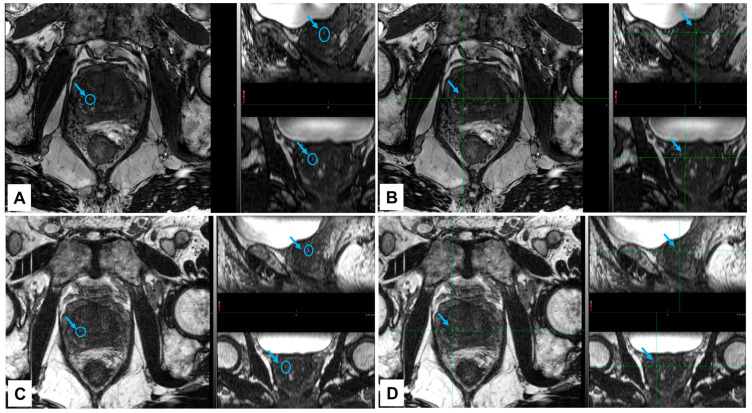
Visibility of NOVA fiducial markers on MR images of a patient receiving proton therapy for prostate cancer. The patient was positioned for MRI, and scans were obtained on a clinical 3.0 T scanner (panels (A,B)) and a 1.5 T scanner (panels (C,D)). MRI scans were acquired with a fully balanced steady state free precession (bSSFP) pulse sequence. NOVA markers were visualized as positive signal on both the 3.0 T images (panels (A,B); blue circles and blue arrows) and the 1.5 T images (panels (C,D); blue circles and blue arrows). Included in each panel are an axial image (acquired with a 15 cm field-of-view and 1.2 mm slice thickness) and two reformatted images (in the sagittal and coronal planes).
